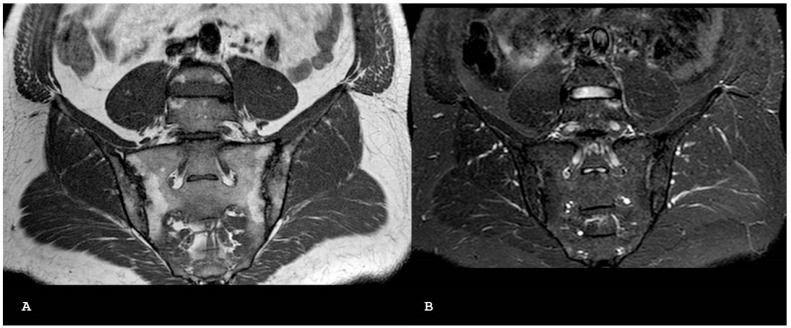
Oblique coronal T1-weighted imaging (A) demonstrates bony irregularity with multiple subchondral erosions on both the sacral and iliac sides and pseudo-widened appearance of both sacroiliac joints. New fat metaplasia in the subchondral bone marrow of the sacral bone is also evident. These findings are suggestive of chronic sacroiliitis. Oblique coronal short tau inversion recovery (B) demonstrates the non-active involvement of both sacral–iliac joints.
